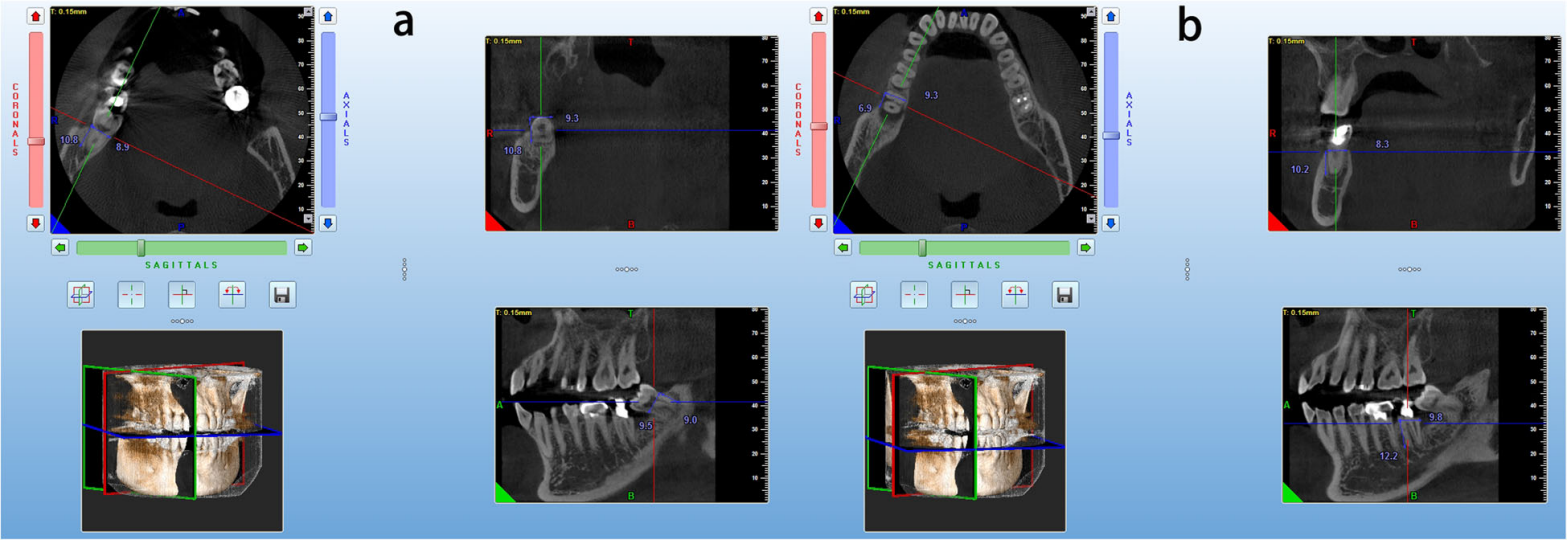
The measurements of the CBCT for preoperative evaluation. a the measurements of the mesio-distal and bucco-lingual dimension and height of the donor tooth at three different axes. b the measurements of the mesio-distal and bucco-lingual dimension and height of the recipient site at three different axes
